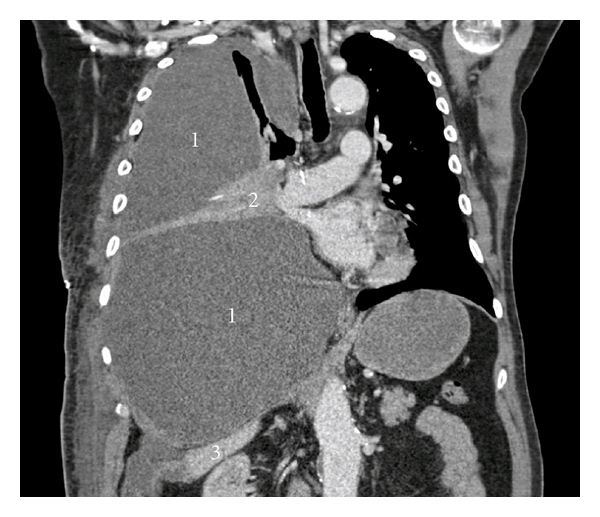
Coronal CT scan image demonstrating the hemothorax (1) with atelectasis of the right lung (2), mediastinal shift to the left, and downward displacement of the liver (3), but no visible intrapulmonary lesions or other signs of malignancy.
